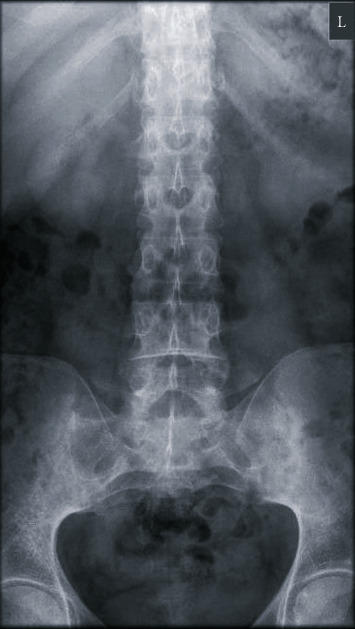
Plain film radiograph of the patient. Anteroposterior plain-film radiograph of the patient performed prior to his initial presentation. Significant sacroiliitis is seen with sclerosis and partial ankylosis of the sacroiliac joints demonstrated bilaterally.
